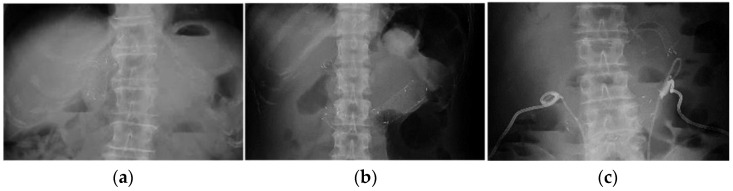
X-ray images of type 2 obstruction in the SEMS cohort (*n* = 3). (a) Endoscopic biliary self-expandable metal stent placement had already been performed before the placement of a self-expandable metal stent for malignant afferent loop obstruction. (b) Simultaneous endoscopic biliary and duodenal self-expandable metal stent placement. (c) Endoscopic ultrasound-guided hepaticogastrostomy was performed a few days after duodenal self-expandable metal stent placement.
